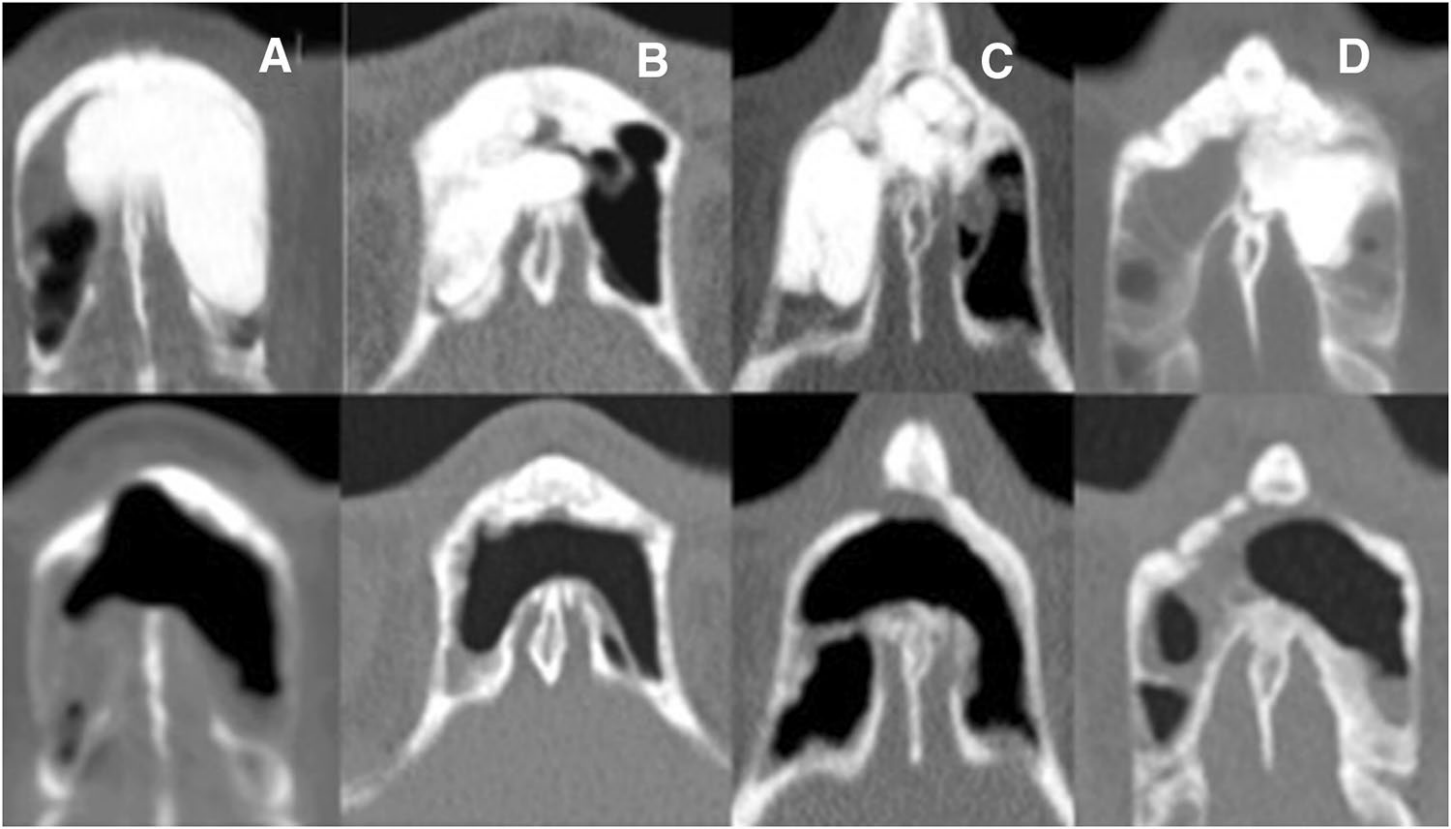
a–d CT axial views of patients with osteomas with compact component at the OG before and after surgery. Note the presence of postoperative osteoneogenesis in patient D
